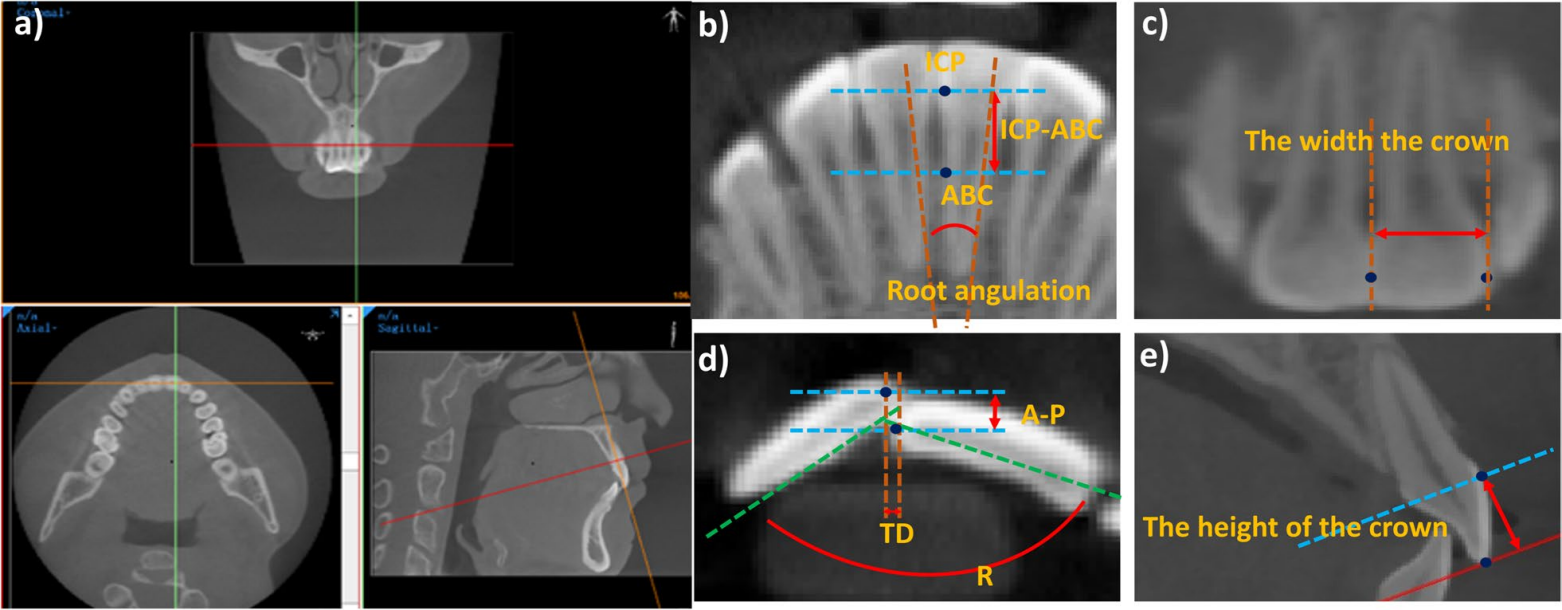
Adjustment and measurement of CBCT images. a On the sagittal plane, adjust the positions of the orange and red lines on the central incisor, and rotate the orange line to make it parallel to the long axis of the tooth. b–c On the coronal plane, adjust anteriorly and posteriorly until the pulp cavity of the central incisor is centered, to obtain the maximum coronal view of the central incisor. Locate ICP and ABC on this plane, and measure ICP-ABC, root angulation crown width. d Keeping the orange line parallel to the long axis of the tooth, adjust on the horizontal plane until the incisal edge is exposed, and measure A-P, TD, and R on this plane. e On the sagittal plane (where the red line is perpendicular to the long axis of the tooth), adjust until the pulp cavity of the central incisor is centered, and measure the crown height
